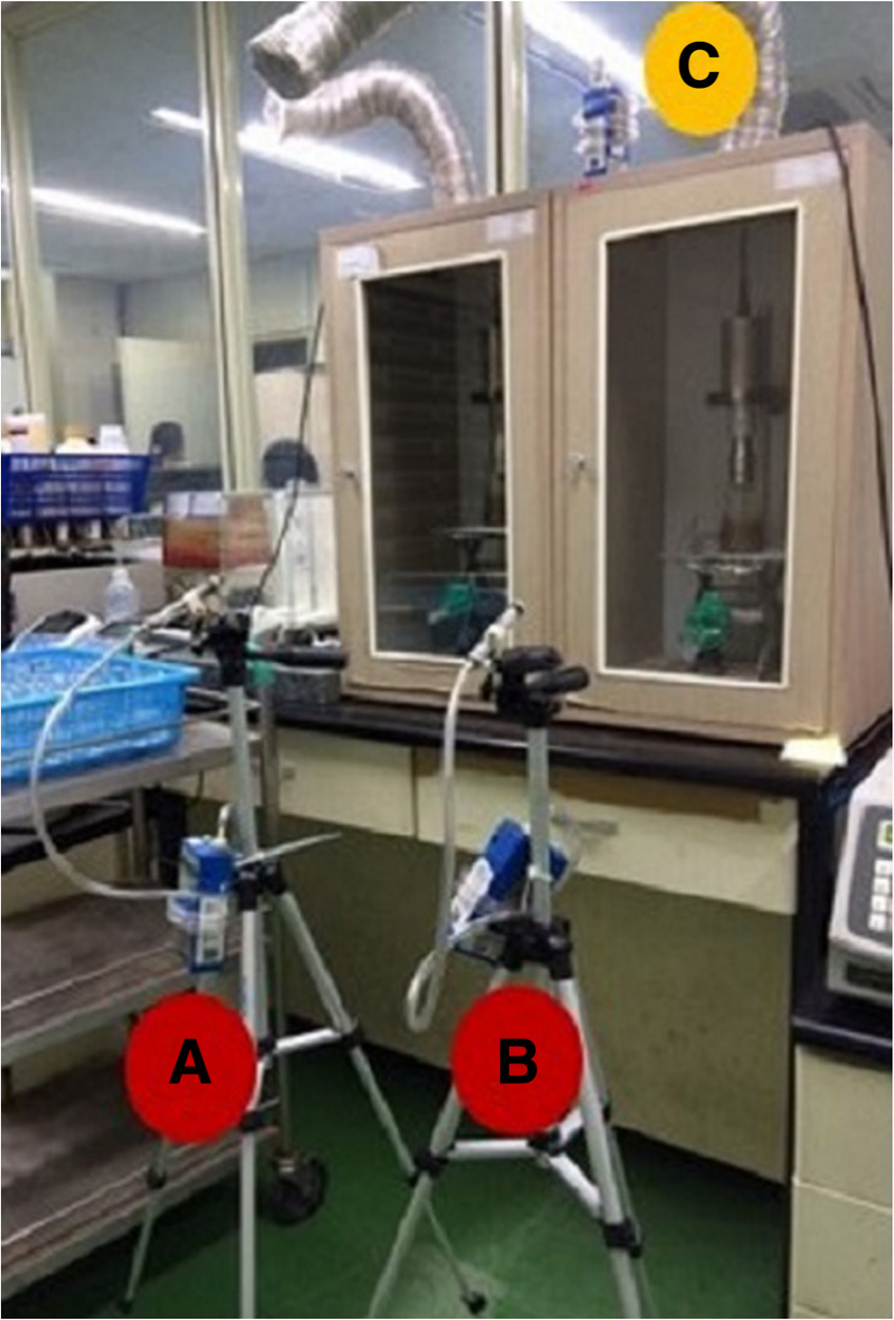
Site of exposure reenactment (Two ultrasound-assisted extractors are located inside separate hood chamber). Measurement position a. In front of Extractor 1. Measurement position b. In front of Extractor 2. Measurement position c. Above the two Extractors
